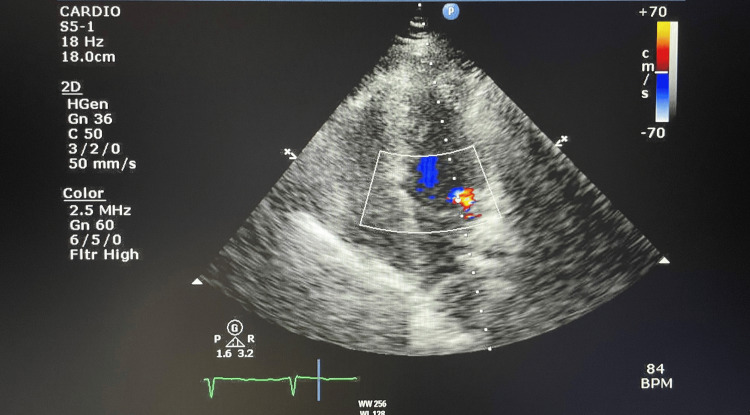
Echocardiography of the patient. Echocardiogram revealing mild mitral regurgitation with slight prolapse movement.
